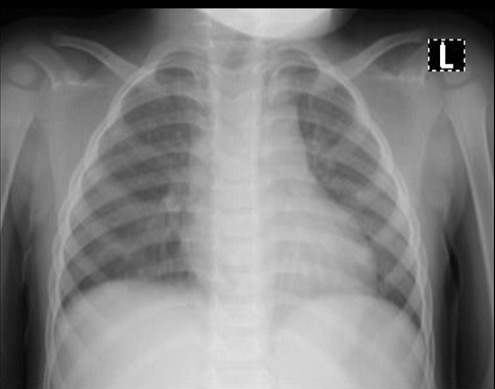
Patient one chest radiograph showing bilateral patchy opacities seen in both lung fields
